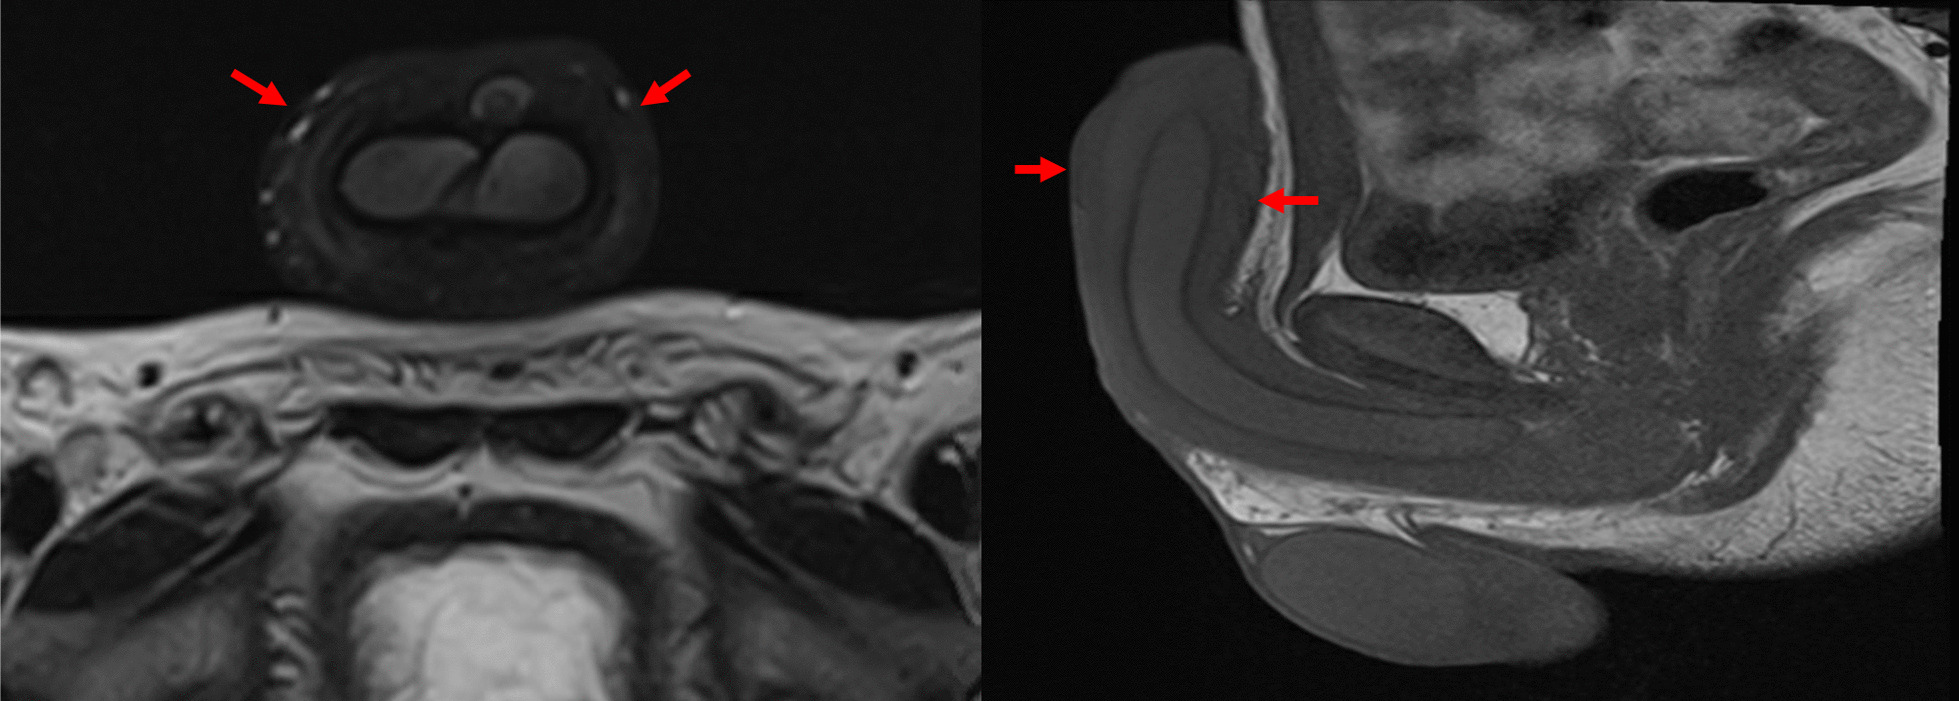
Pelvis magnetic resonance imaging showed non-specific subcutaneous soft tissue thickening surrounding the penis (see the red arrows). No evidence of collections/lymphatic malformation within the pelvic region
